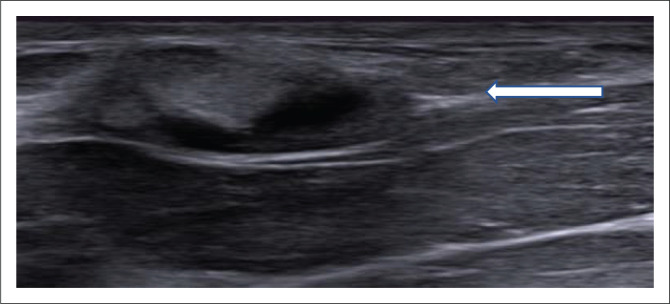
Greyscale ultrasound image of a 50-year-old female initially diagnosed with estrogen receptor/progesterone receptor+ invasive ductal carcinoma (IDC), grade II with an intraductal component. Four months post-BCT, the patient developed a mass. The ultrasound image shows an anechoic parallel mass with a non-dependant soft tissue component and mixed posterior acoustic change. The corresponding mammogram showed a soft tissue mass. The final BIRADS classification was 4B and the patient underwent stereotactic biopsy which confirmed fat necrosis.
